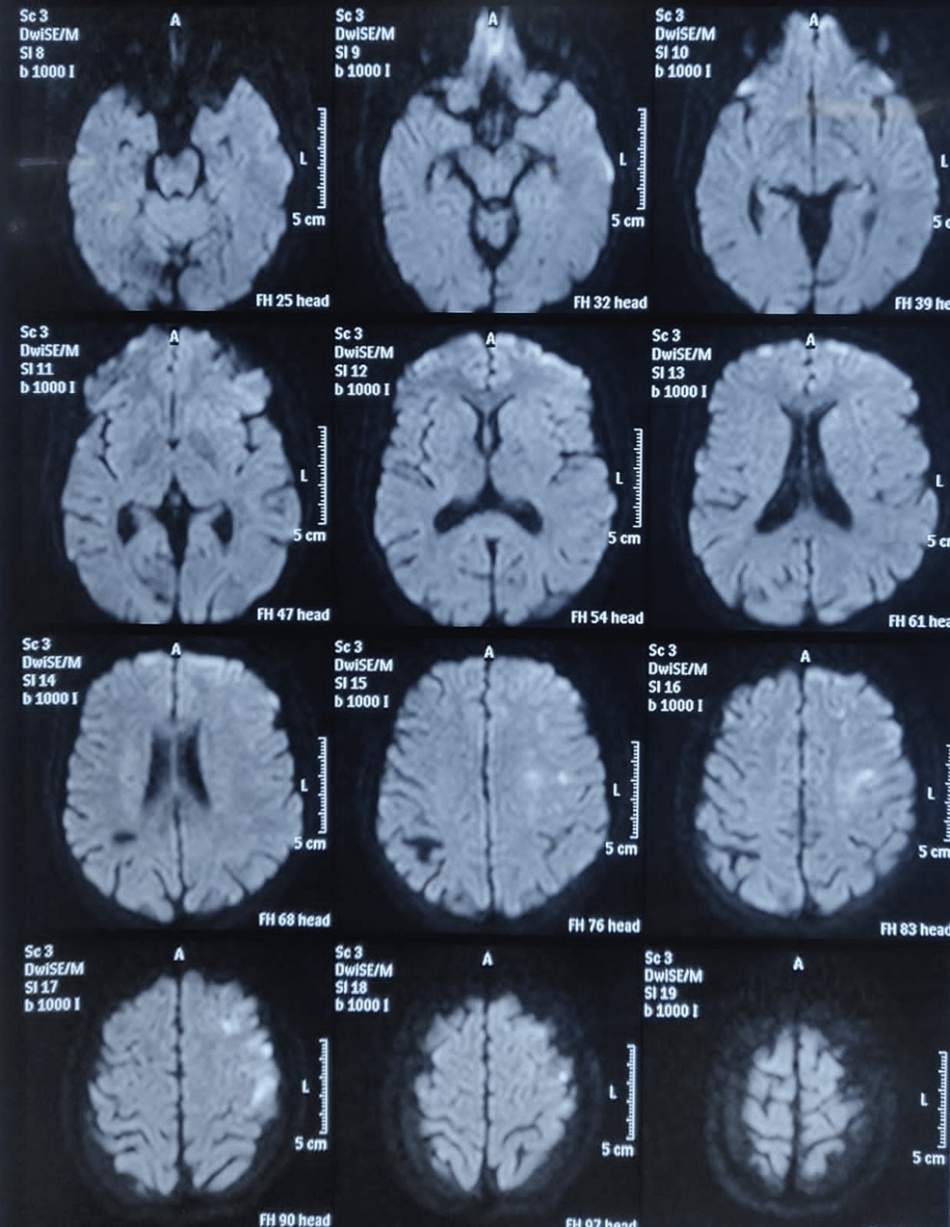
Magnetic resonance imaging (MRI) of the brain showing multiple restrictions in the left frontal as well as high parietal region suggestive of multiple non-hemorrhagic infarcts
